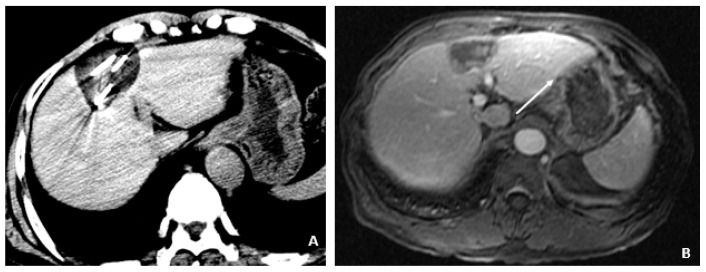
Cryoablation of the segment 4A HCC (April and May 2009). (A) The CT image through the region of the segment 4A HCC shows the cryoprobes and formation of the surrounding ice ball that accumulates as freezing cycles are applied to ablate the lesion. (B) A delayed postcontrast T1-weighted fat-suppressed image 1 month later shows little to no apparent enhancement in the ablated lesion (arrow).
